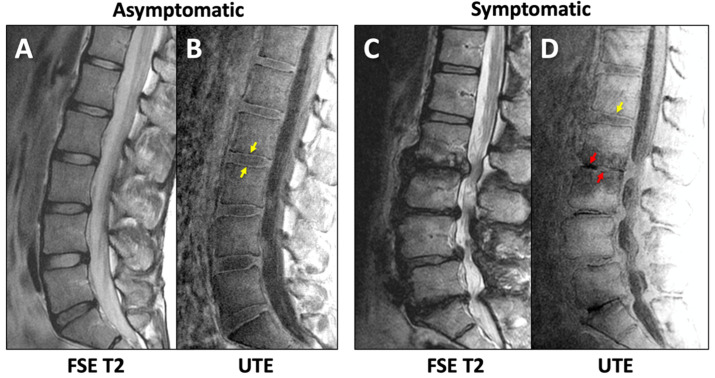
Sagittal lumbar imaging of asymptomatic (A,B) and symptomatic (C,D) subjects using FSE T2 (A,C) and UTE (B,D) techniques. Normal (yellow arrows in (B,D)) and abnormal (red arrows in (D)) morphology of the DVJ can be seen in the UTE MR images.
